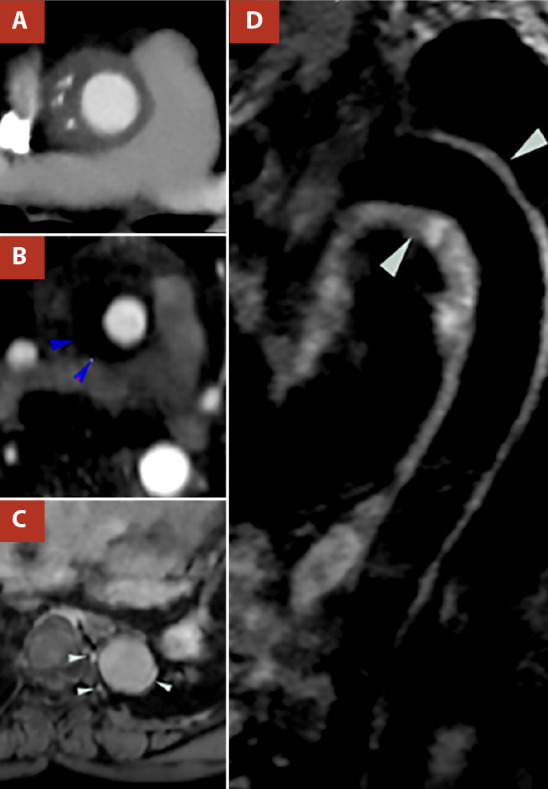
(A)Pre-treatment contrast Computed tomography angiography reformat showed concentric lesion hypoattenuated with 77 HU and thickening of 1.2 cm at the proximal ascending thoracic aorta. (B) Pre-treatment post-contrast resonance angiography showed a thickened ascending aorta wall (13 mm) with a hypointense signal. (blue arrow). (C) Pre-treatment T1w sequence MR in the same patient demonstrated enhancement of the descending aortic wall after administration of intravenous contrast (white arrow). (D) Pre-treatment Double inversion recovery fat sat T2w sequence MR in the same patient at different levels shows a diffuse hyperintense lesion of the aortic wall compatible with periaortic edema (white arrow).
